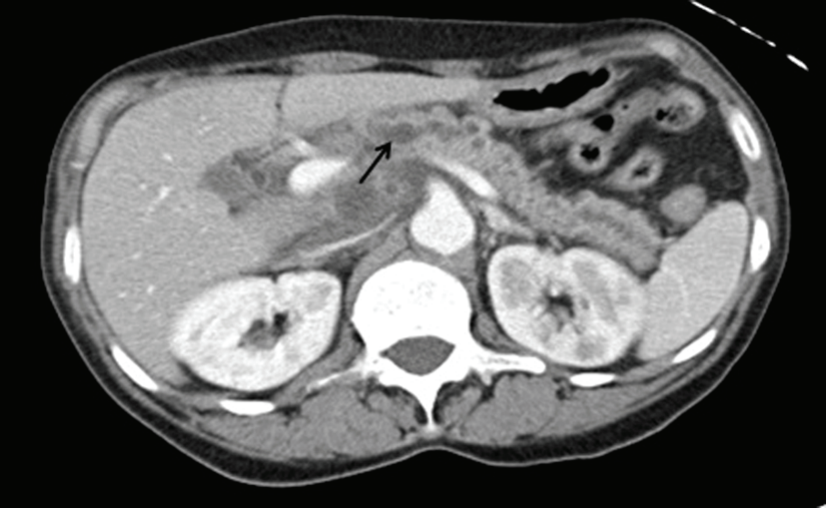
Contrast-enhanced CT scan of the abdomen reveals dilated pancreatic duct possibly filled with blood.
